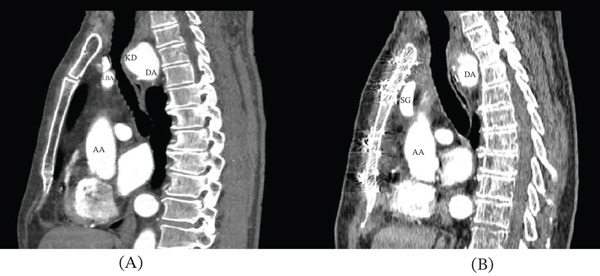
Arterial phase of the contrast‐enhanced CT scan in the left sagittal view (A) before and (B) after the procedure. AA, ascending aorta; DA, descending aorta; ES, esophagus; IV, innominate vein; KD, Kommerell’s diverticulum; LBA, left brachiocephalic artery; LCCA, left common carotid artery; LSA, left subclavian artery; SG, synthetic graft; RCCA, right common carotid artery; RSA, right subclavian artery; TR, trachea.
